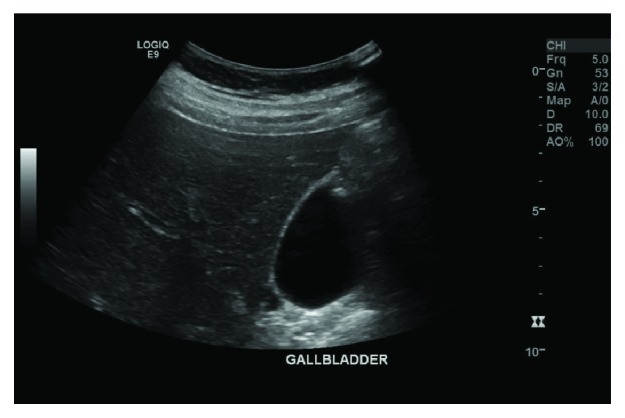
US of the abdomen. Gallbladder with no sonographic evidence of cholecystitis or cholelithiasis.
